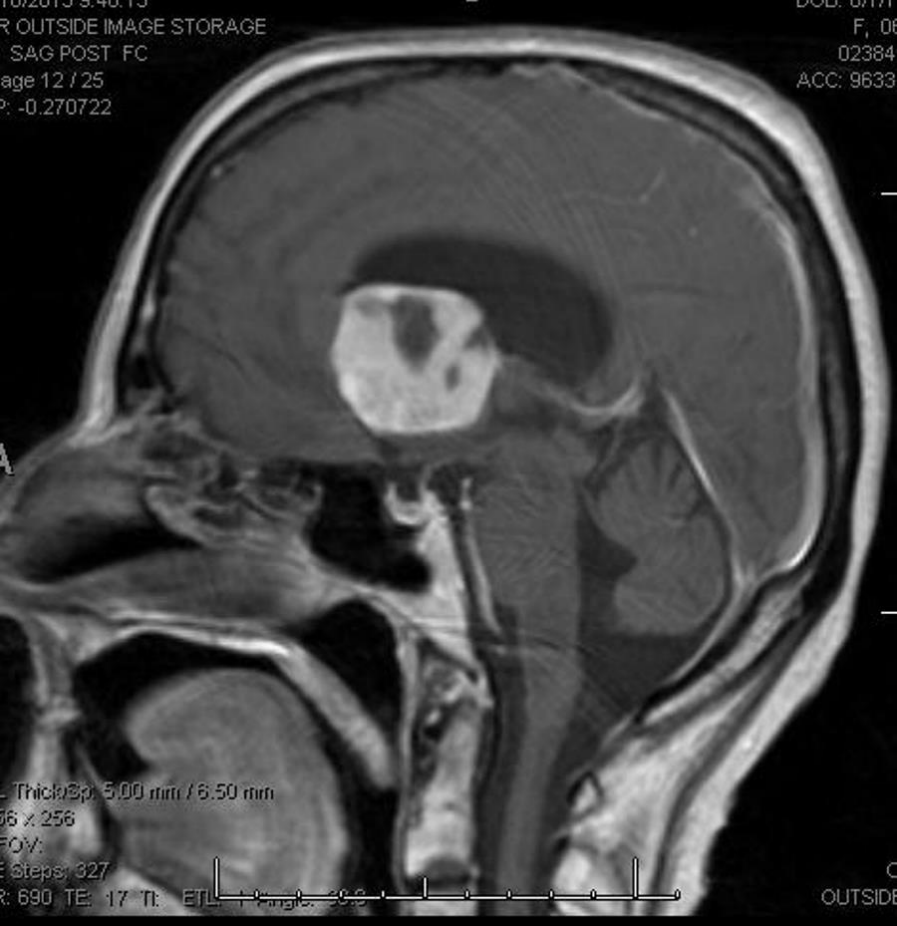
Initial MRI (post-contrast) T1 sagittal post-contrast MRI scan showing mass effect of the lesion on the foramen of Monro.
